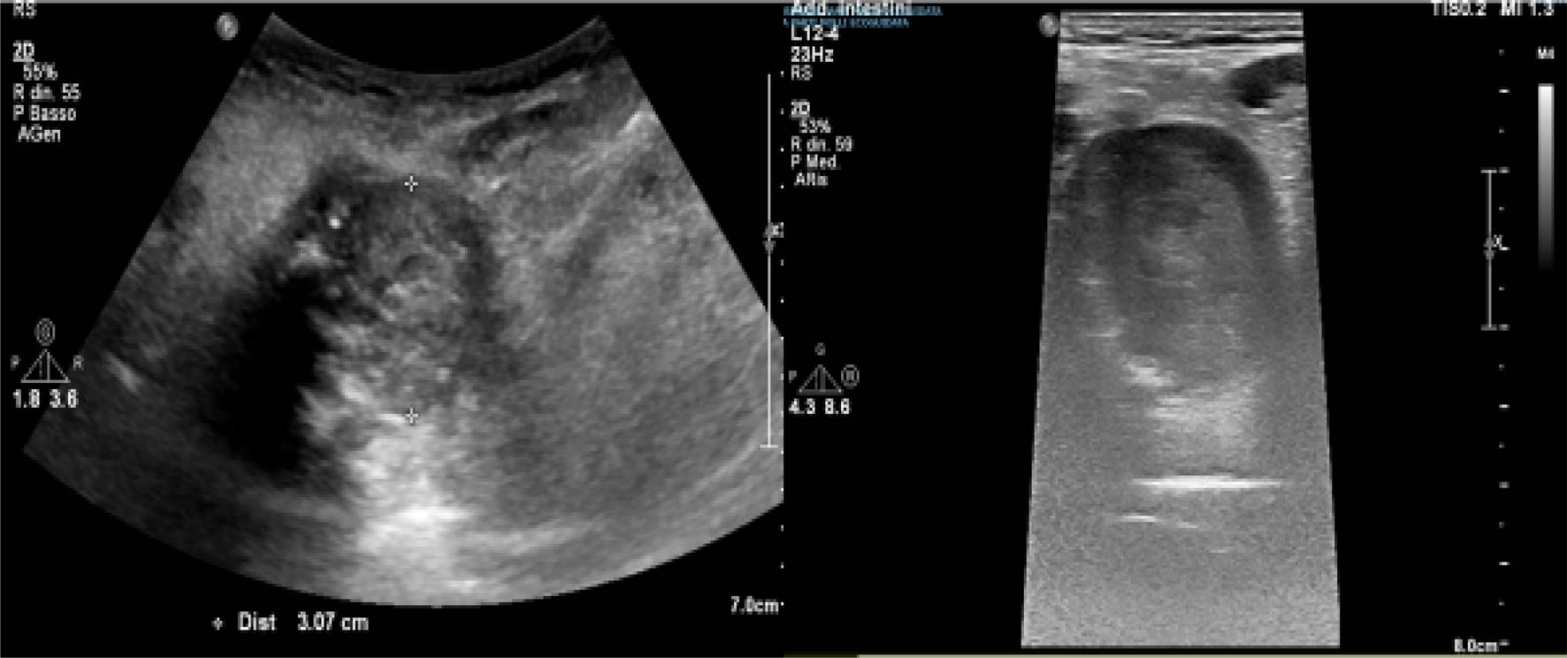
B-mode ultrasound with convex and linear probe in the upper midline of the neck. On the right-side of the pharynx, an oval formation with a non-homogeneous echostructure measuring approximately 30 mm is visible
